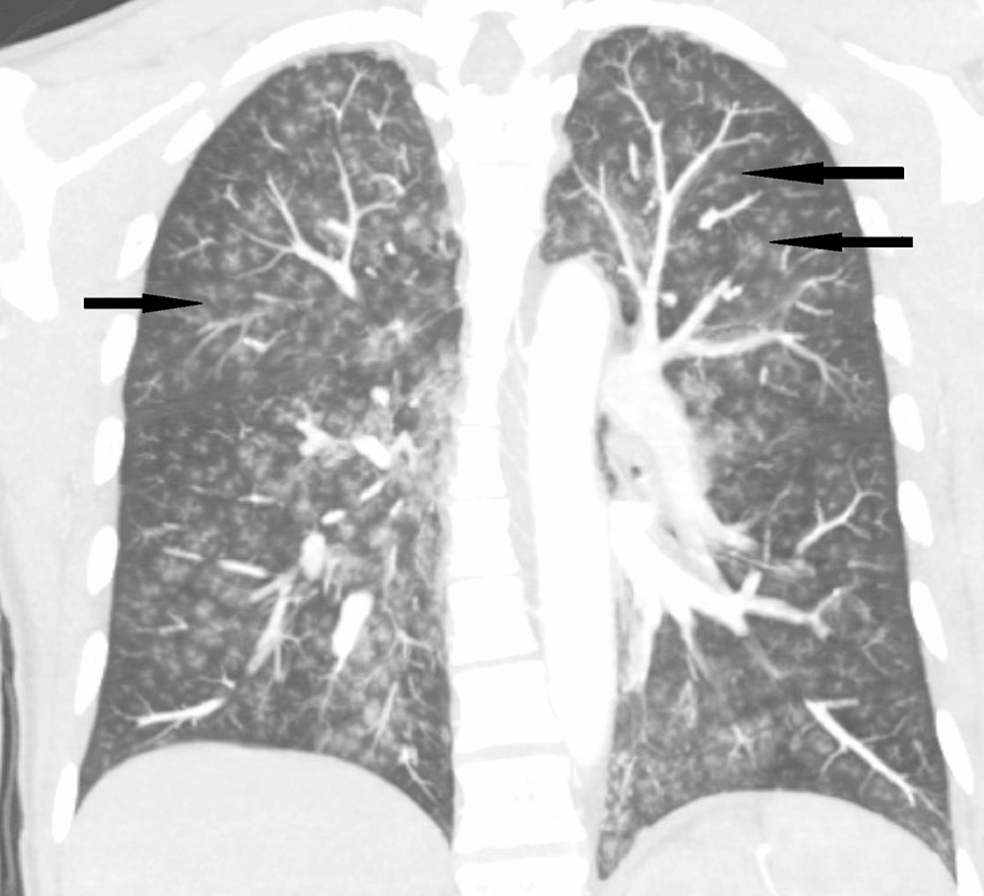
Coronal view of CT angiogram of the chest showing bilateral extensive nodular opacities (examples shown by arrows)
